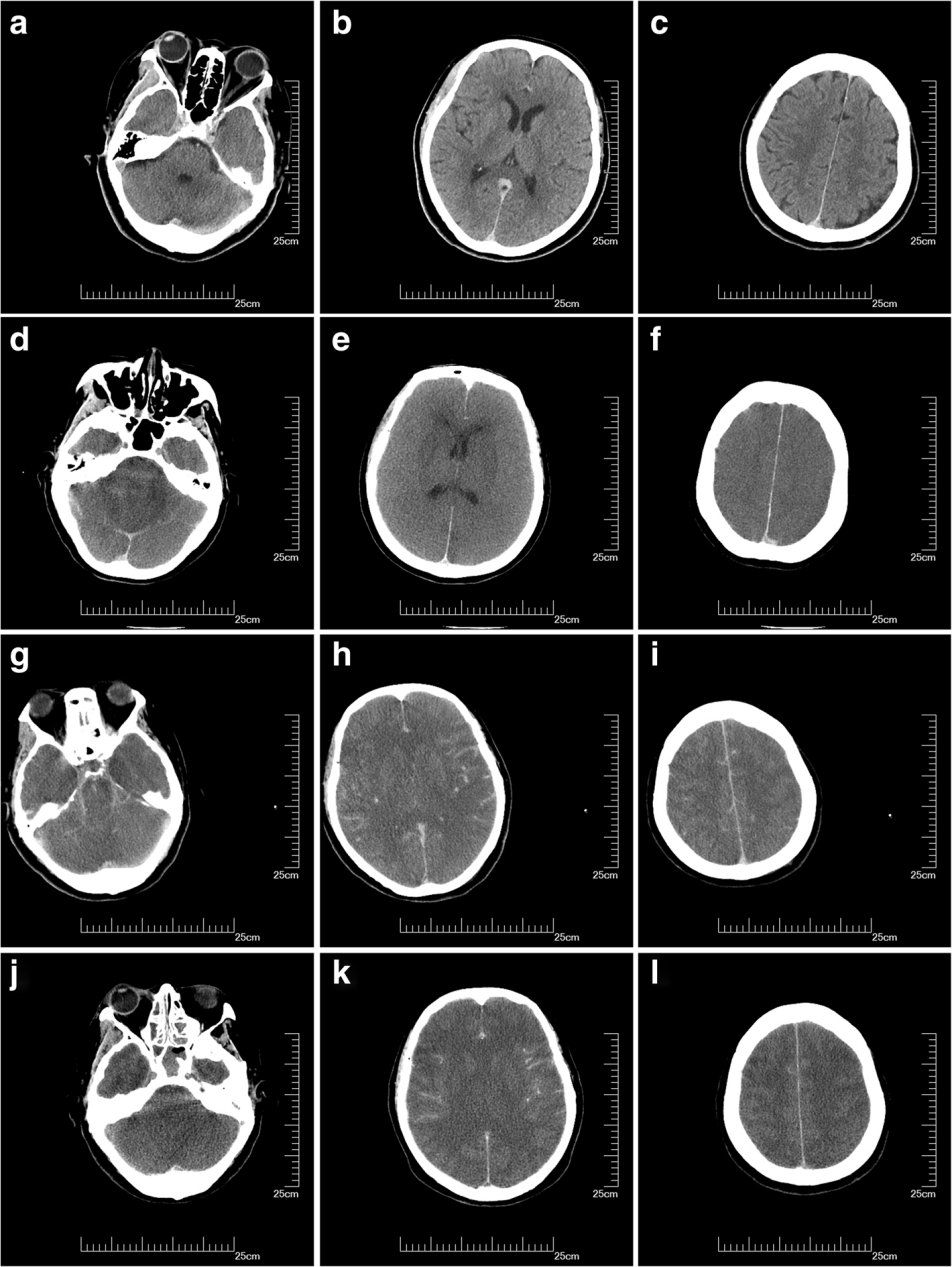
Cerebral CT immediately after DSA did not indicate any obvious abnormal sign (a, b, c); 2 days after the procedure, a repeat cerebral CT revealed diffuse cerebral oedema, loss of grey-white differentiation, effacement of the cerebral sulci and decrease in cerebrospinal fluid space (d, e, f); 9 days after the procedure, the cerebral CT showed more severe diffuse oedema of the brain, loss of grey-white differentiation, effacement of the cerebral sulci and subarachnoid space, disappearance of the cerebral ventricles, enhancement of the subarachnoid space, and darkened brain tissue in Hounsfield units (g, h, i); 15 days after the procedure, the cerebral CT showed persistent diffuse oedema of the brain with effacement of the cerebral ventricles and sulci, darkened brain tissue in Hounsfield units, and enhancement of the subarachnoid space (j, k, l)
